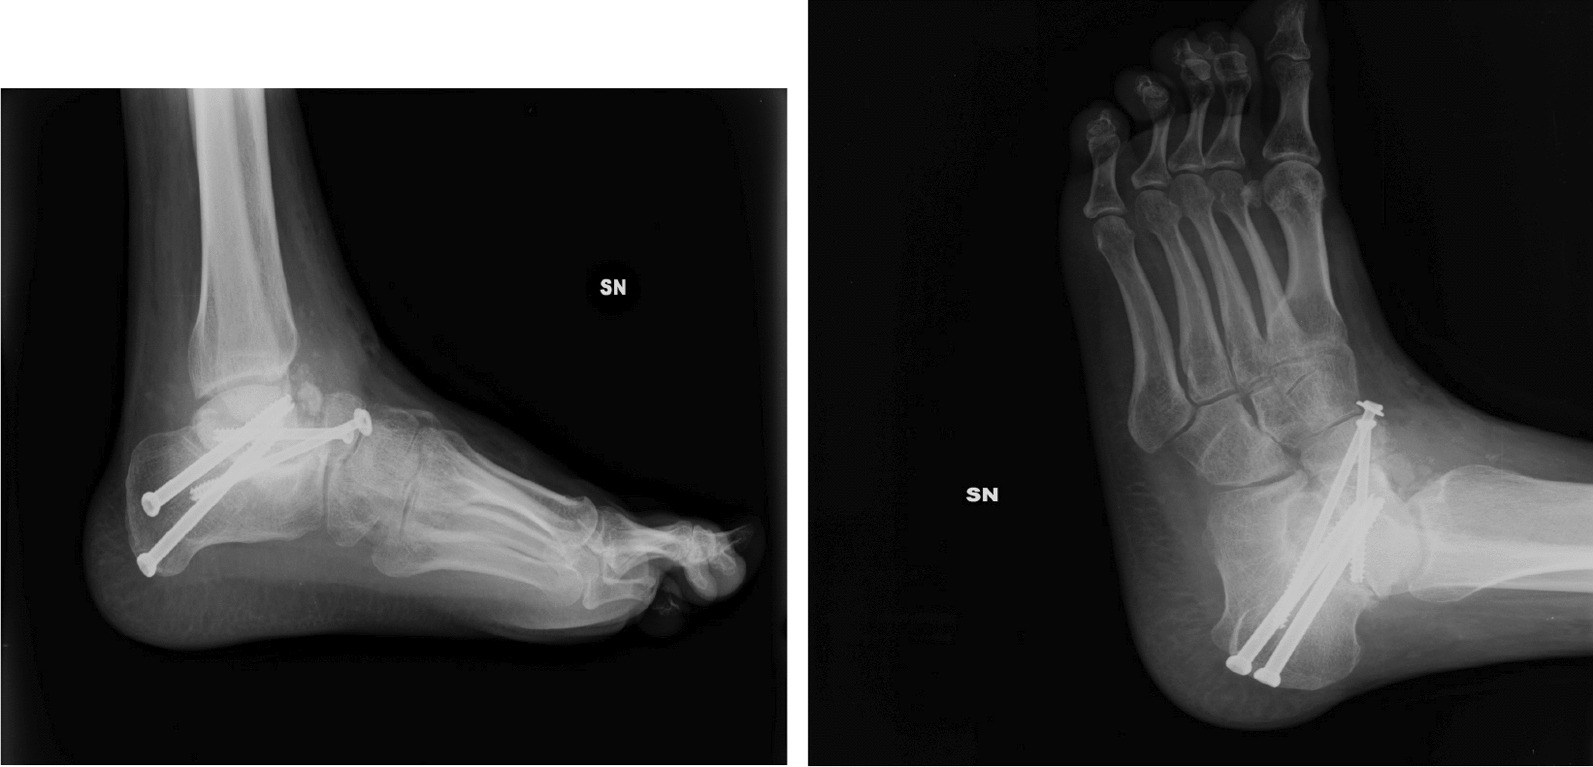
Post-operative x-ray of revision arthrodesis
